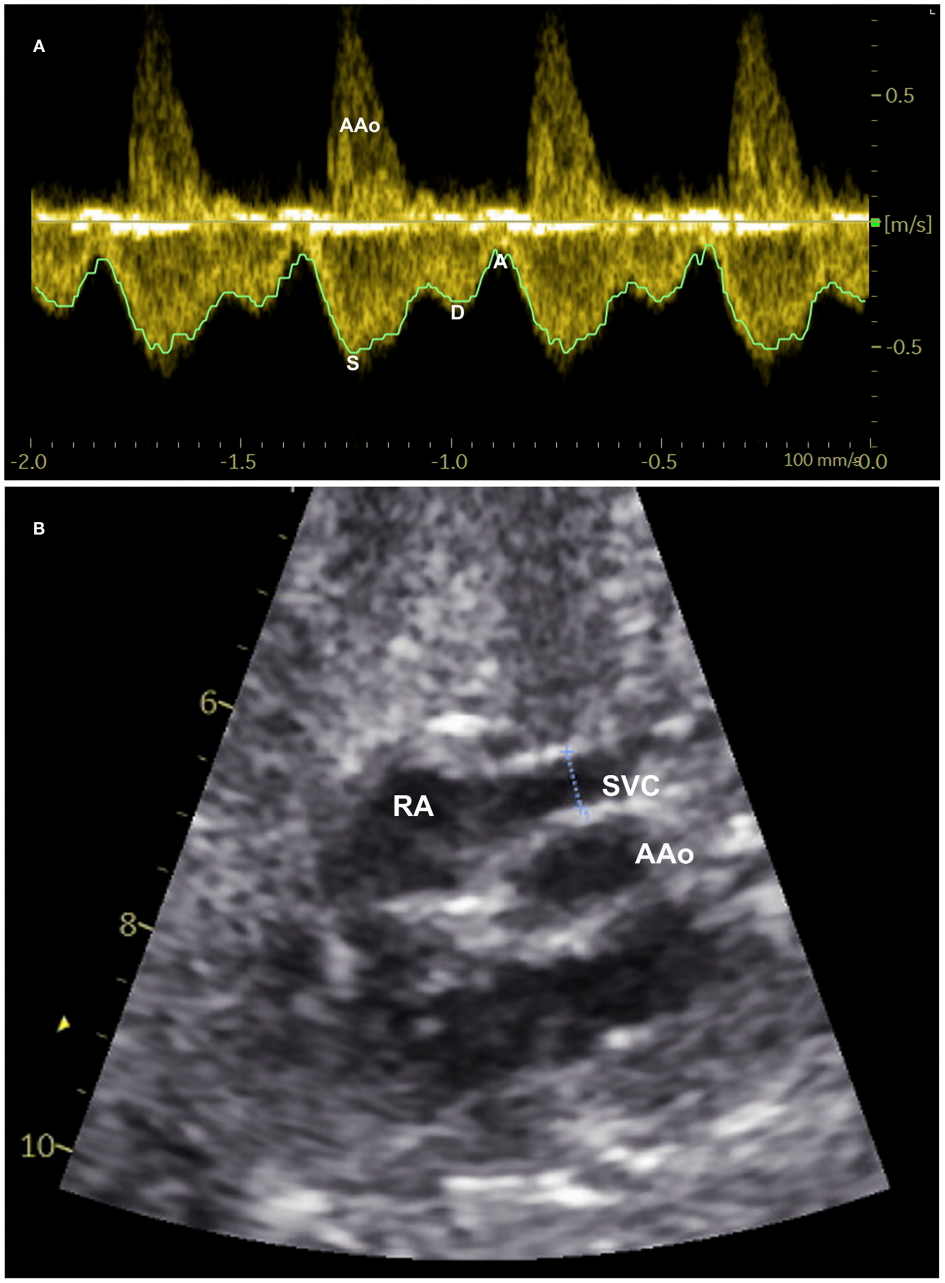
Measurement of superior vena cava (SVC) blood flow: (A) Spectral pulsed-wave Doppler velocity waveforms of the fetal SVC with tracing of maxium velocity envelope over cardiac cycles to measure time-averaged maximum velocity. S-maximum velocity during systole, D-peak velocity during diastole, and A-minimum velocity during atrial contraction. (B) Two-dimensional B-mode ultrasound image of SVC demonstrating measurement of its inner diameter (dotted line between two calipers) shortly above the entrance to the right atrium (RA). AAo-Ascending aorta.
